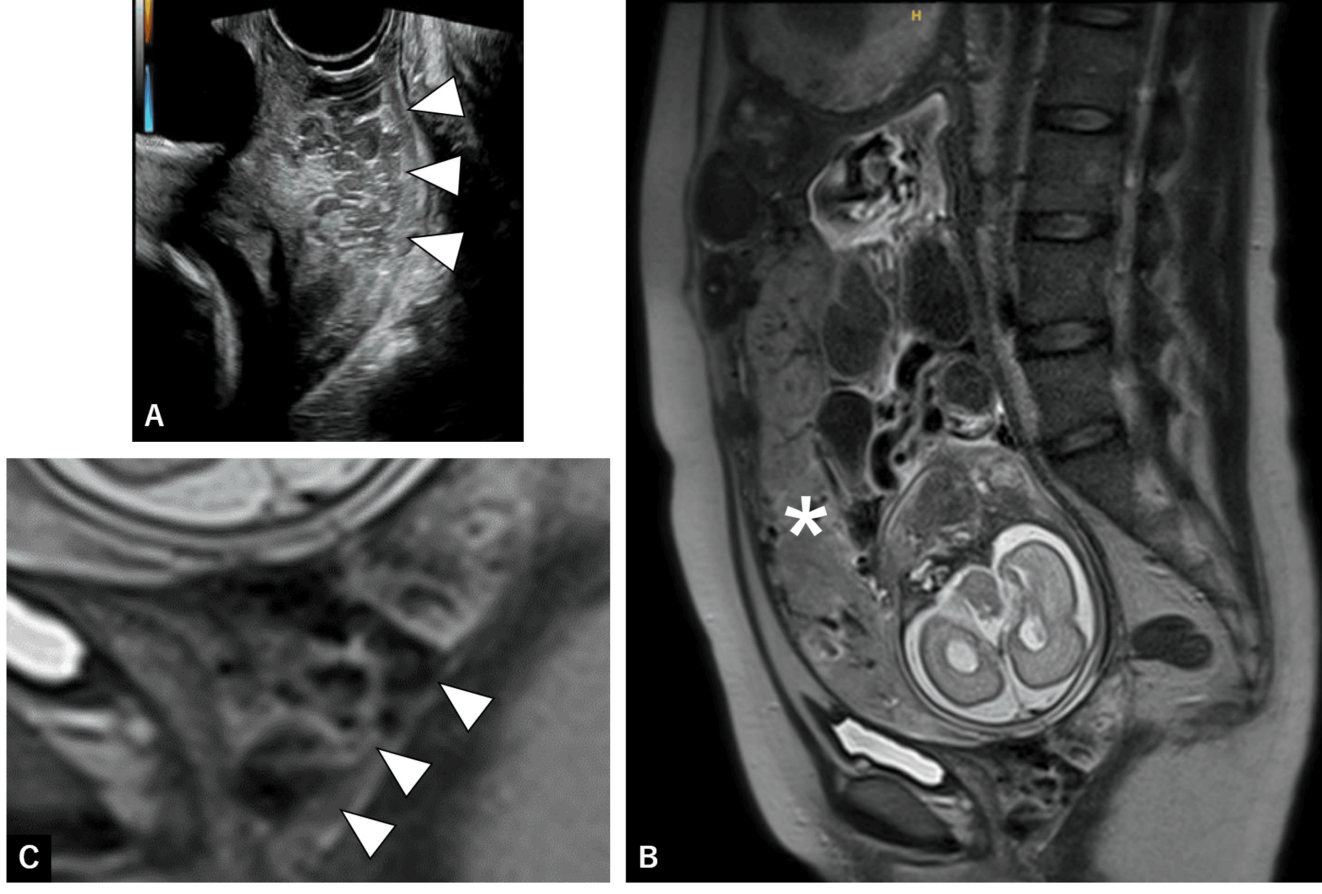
Ultrasound and MRI images (A) Transvaginal ultrasonography at 22 weeks of gestation. Arrowheads indicate dilated vessels within the cervix. (B, C) Magnetic resonance imaging (MRI) at 26 weeks of gestation. (B) T2-weighted sagittal view shows the low-lying placenta (asterisk) attached to the anterior uterine wall. (C) Enlarged image of (B). Note that dilated vessels are replacing the anterior cervical lip.
